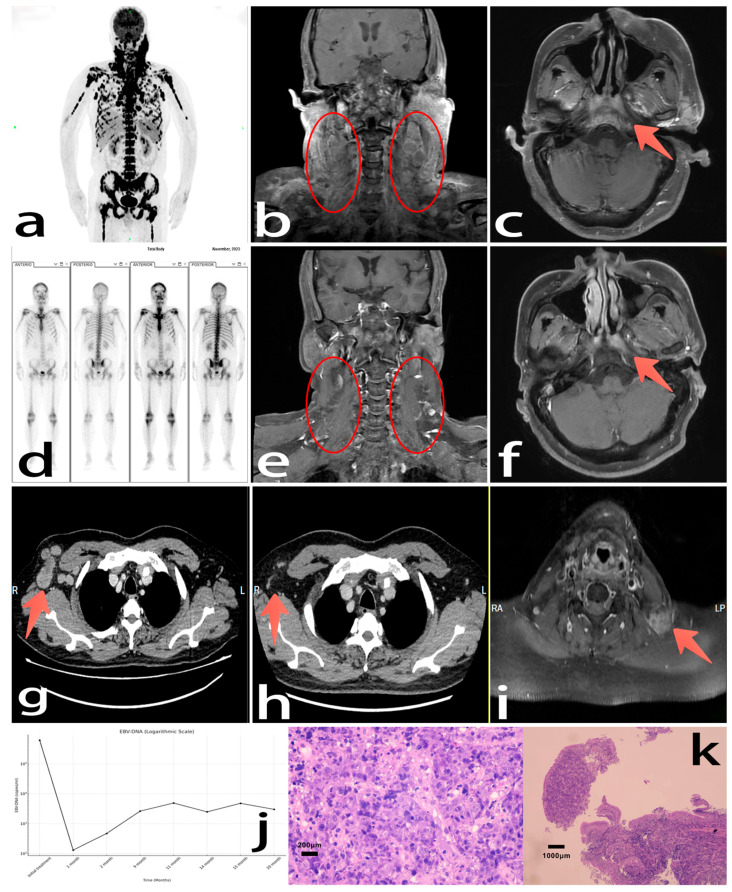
Initial diagnostic imaging: (a) Pre-treatment PET-CT showed NPC with extensive skeletal and nodal metastases. (b,c) Pre-treatment MRI revealed clivus invasion and large-scale fusion of cervical lymph nodes with invasion of muscles and skin. Seven months after two cycles of first-line therapy. (d) SPECT showed complete metabolic resolution of skeletal metastases. (e) Coronal MRI suggested nodal lesions near-complete recession. (f) Axial MRI suggested diminished nasopharyngeal and clivus enhancement. (g) A newly detected axillary lymphadenopathy on CT. Fourteen months after diagnosis and five months after the second treatment. (h) CT showed complete regression of the right axillary lymphadenopathy. (i) MRI showed a new nodal lesion in the left cervical region. (j) EBV-DNA load fluctuations: EBV-DNA copies dropped rapidly after two cycles of treatment, slightly increased, and remained relatively low until the last follow-up date. (k) Pathological results suggested an undifferentiated non-keratinizing carcinoma.
